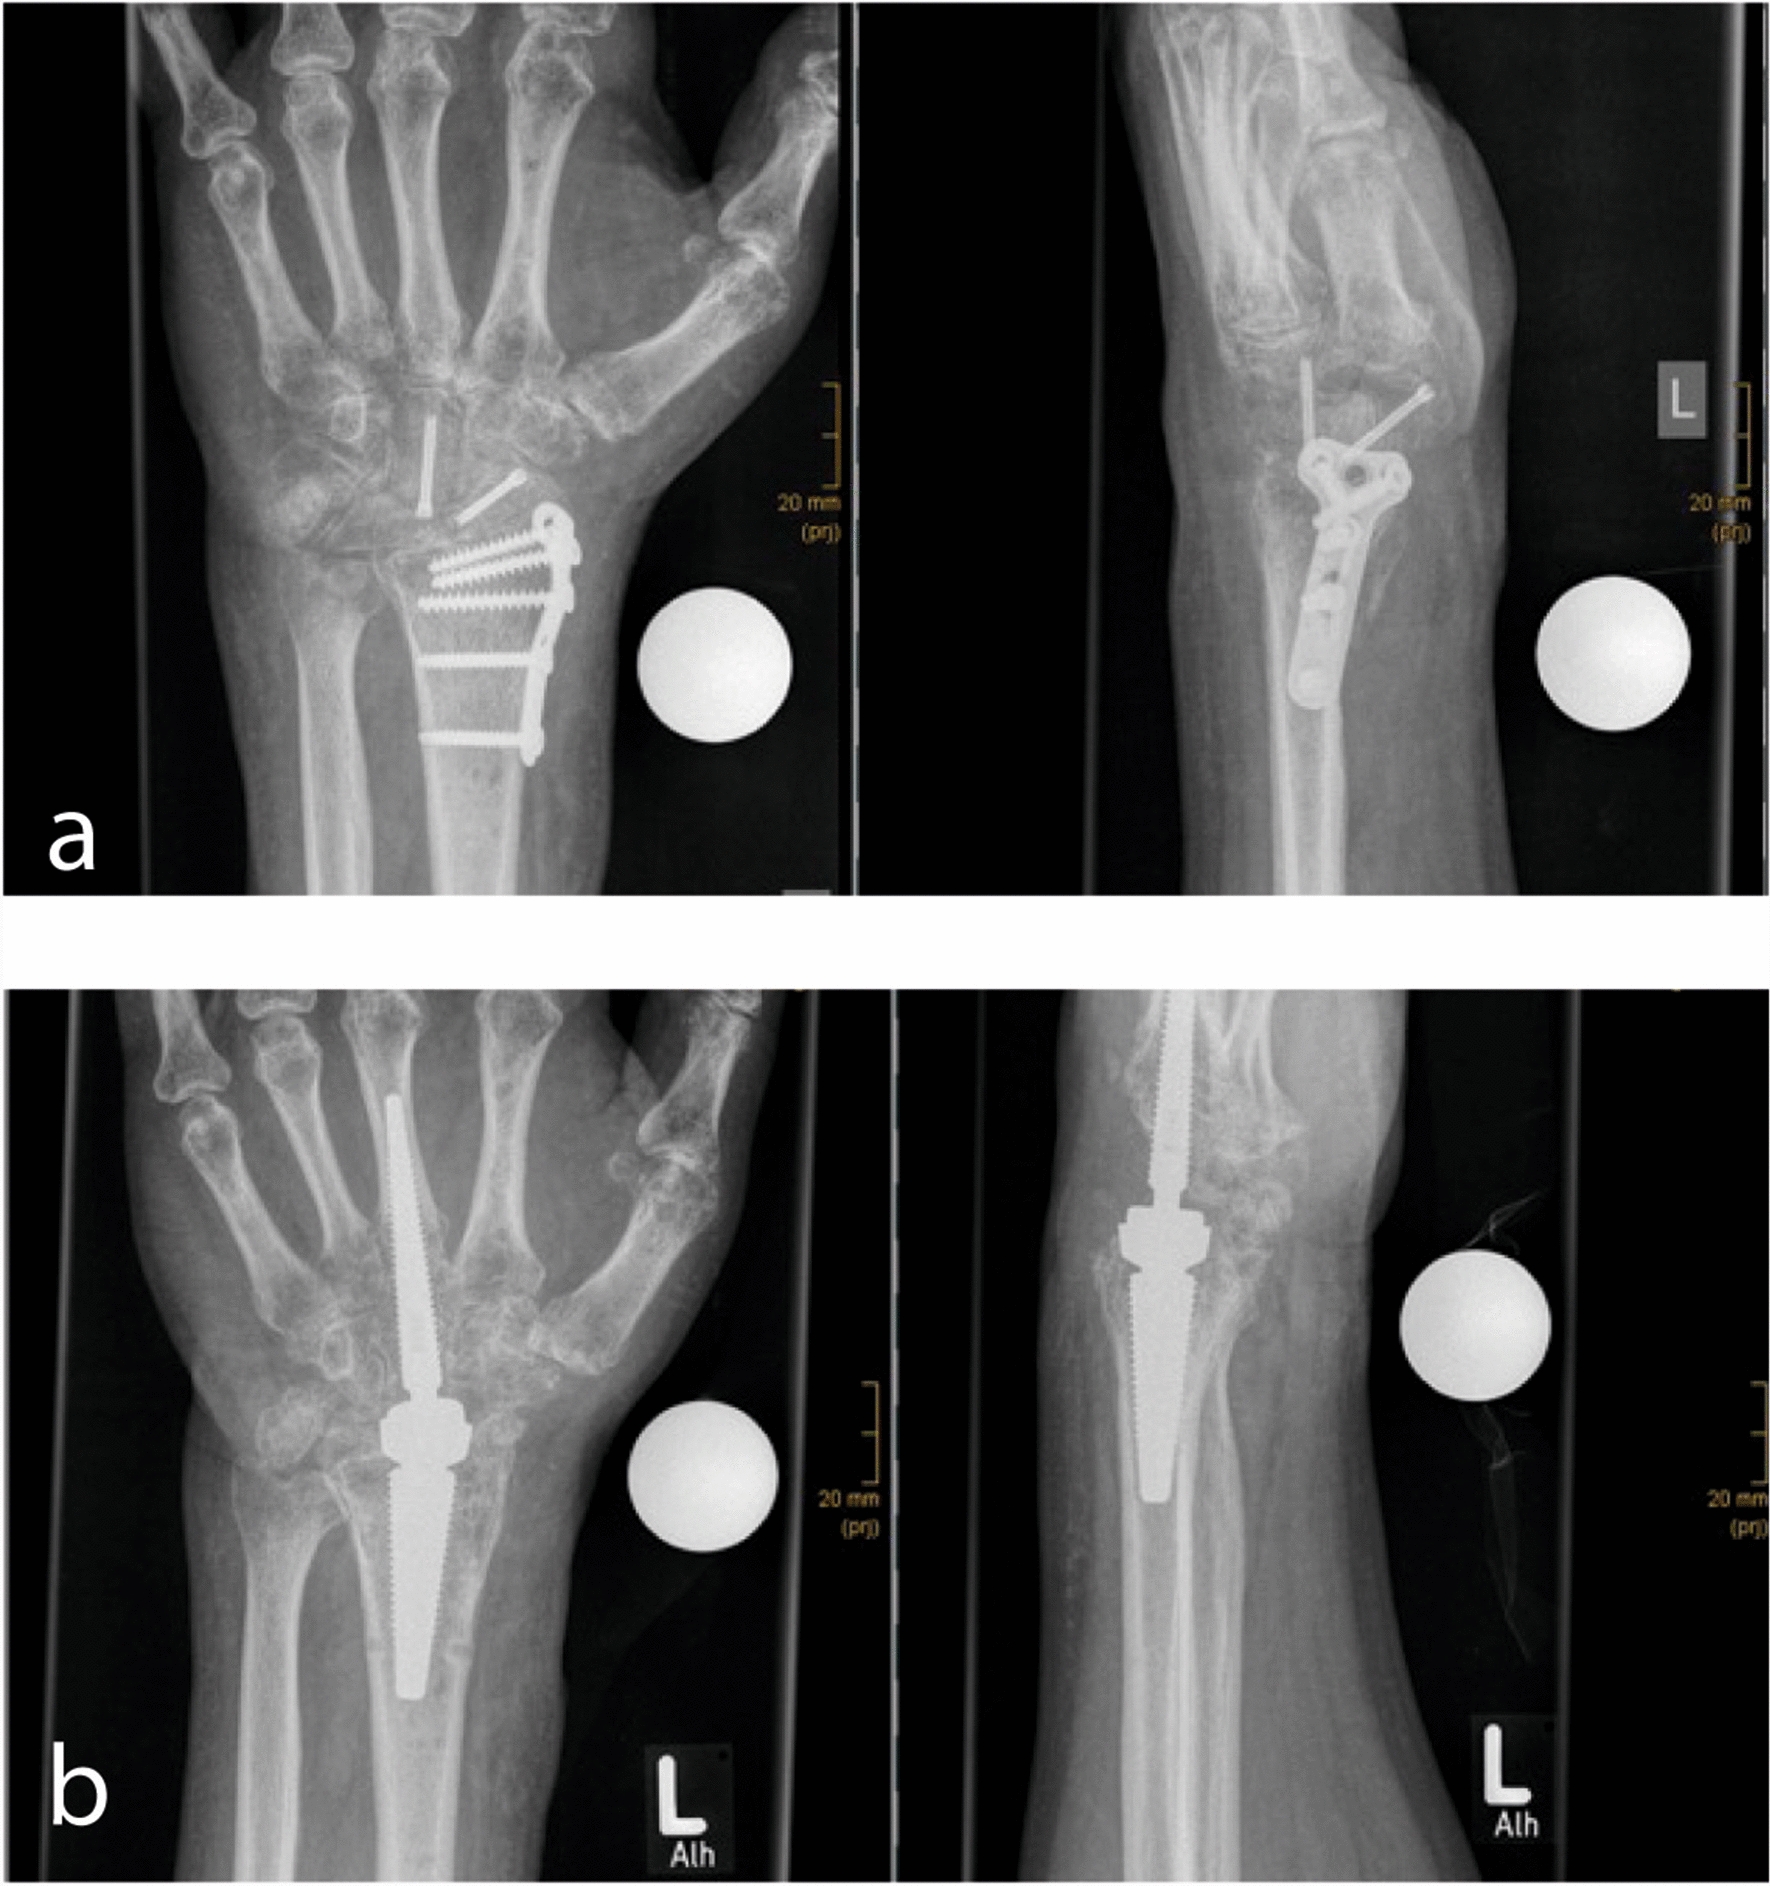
X-rays of the left hand of the patient in the anterior–posterior and lateral views. a Preoperative x-ray. Herbert screws and T-plate of the initial surgeries are still in situ. Advanced mediocarpal and radiocarpal arthrosis of the left wrist. b Preoperative X-ray with sign of heterotopic ossification in the joint gap
